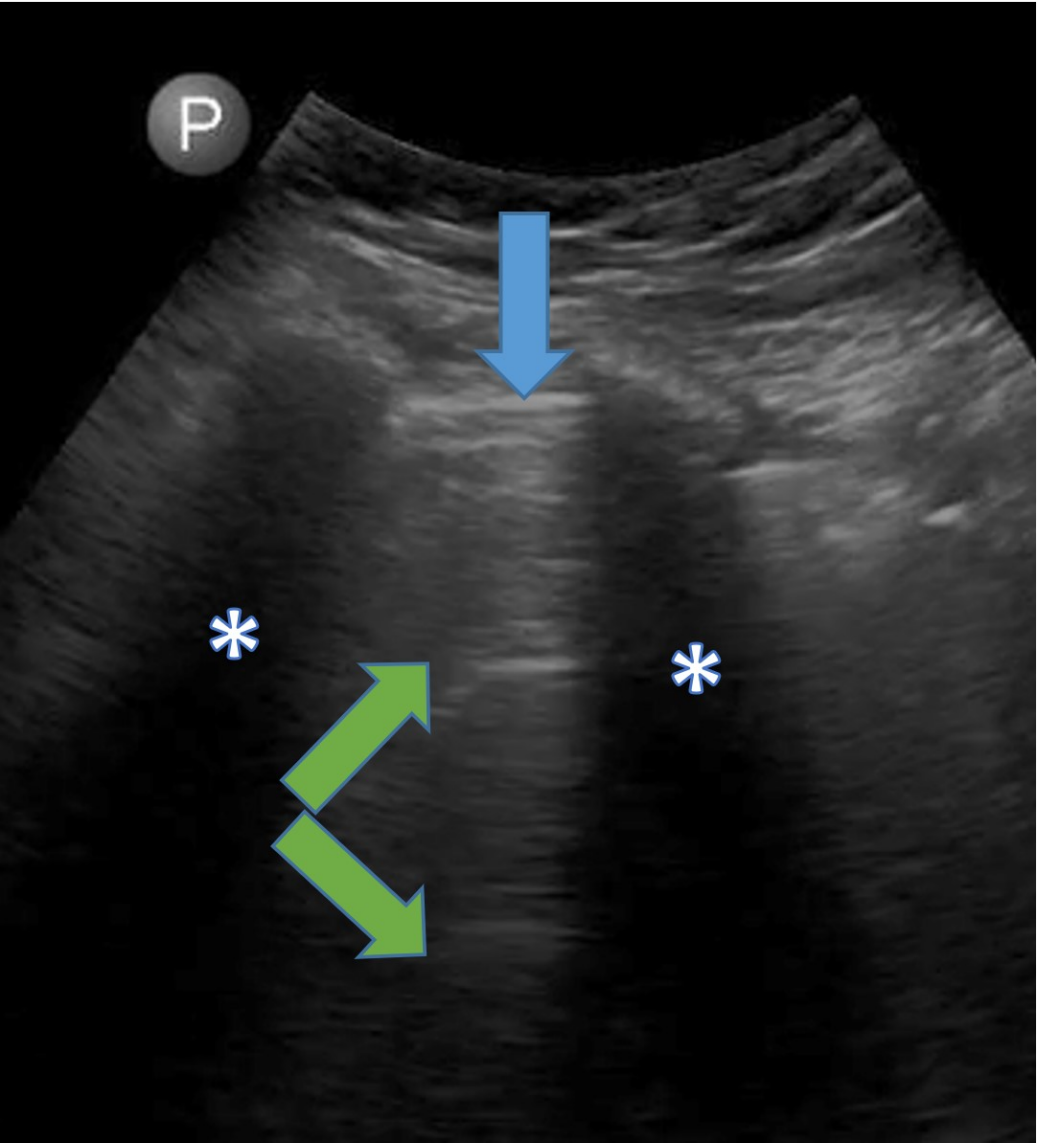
Lung ultrasound image obtained using a curvilinear probe. Blue arrow shows the pleural line,Green arrows show A-lines. Asterisks indicate rib shadows.
